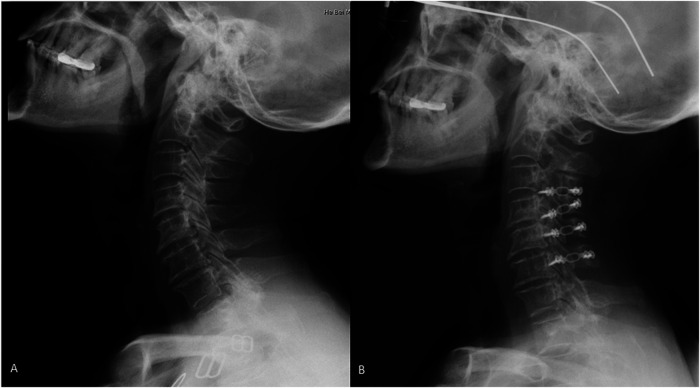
Posterior cervical surgery was performed to release the compression. Lateral x-ray of cervical spine was taken in a male patient with OPLL at preoperative and postoperative. A is preoperative, B is postoperative.
